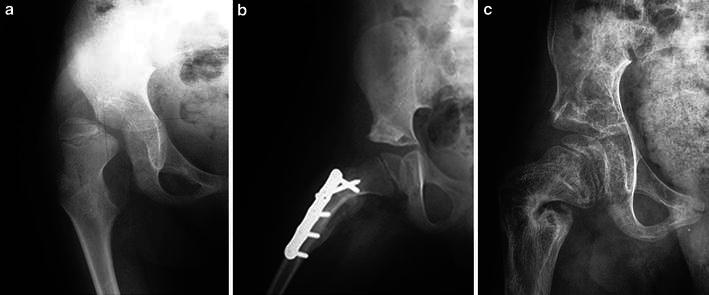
a Pre-operative X-rays of a 6-year-old boy with neurologic hip dislocation. b Postoperative result after Dega procedure and femoral shortening, varus and derotation osteotomy. c Long-term follow-up
